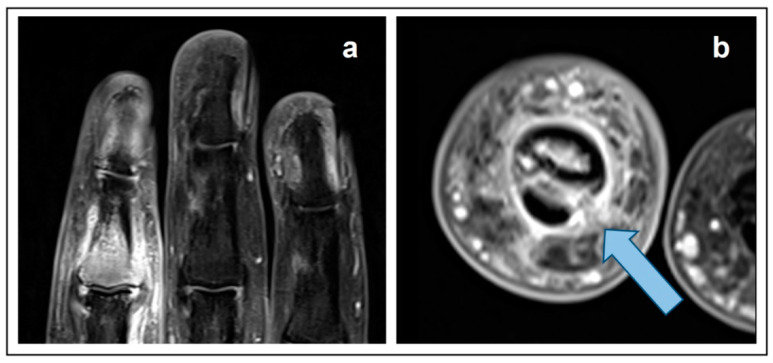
T1-weighted fat-saturated, contrast-enhanced MRI. (a) coronal view. (b) axial view.
